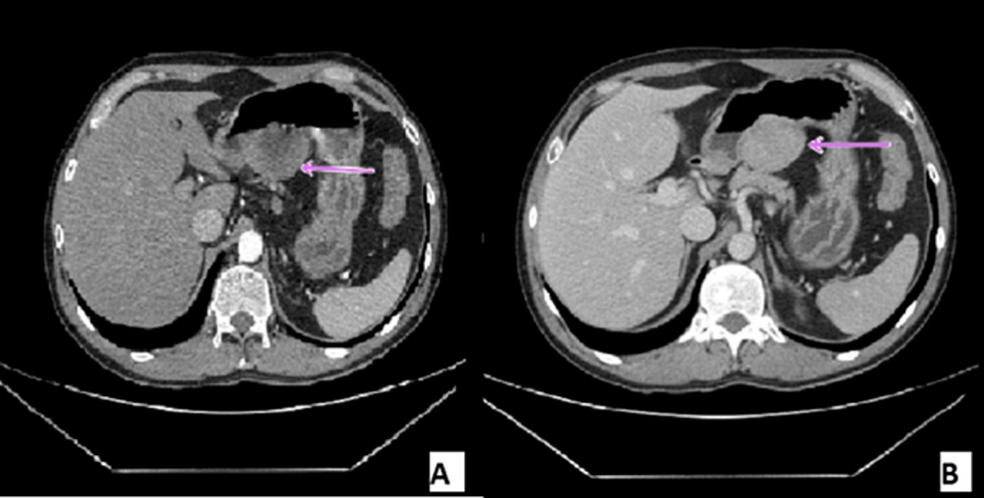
Contrast-enhanced CT scan shows a round homogeneous lesion (arrow) arising from the lesser curvature of the stomach showing homogenous enhancement in the venous phase A - arterial phase image, B - venous phase image
